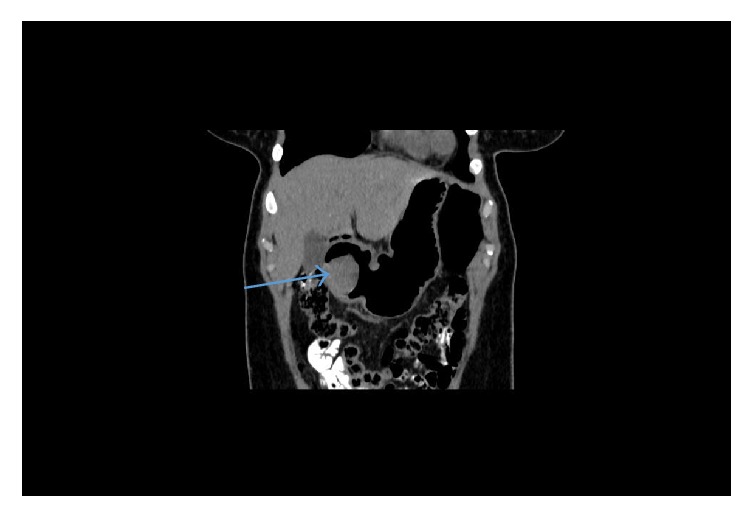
Coronal section of CT scan of abdomen, showing gastric mass near pylorus with no local invasion outside the gastric mucosa.
